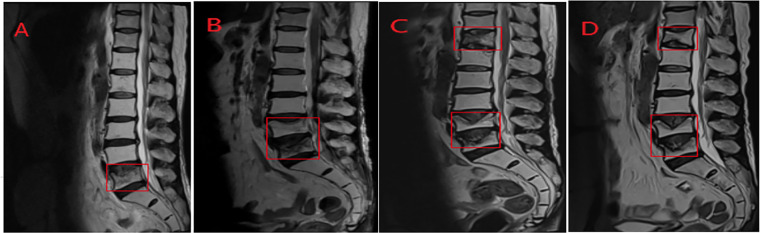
Lumbar Spine MRI scanning of the patient. (A) Compression Fracture of the L5 Vertebral Body. (B) Compression Fracture of the L4 and L5 Vertebral Bodies. (C) Compression Fracture of the T12, L4, and L5 Vertebral Bodies. (D) Bone destruction in the sacrum and the posterior part of bilateral iliac bones, and Compression Fracture of the T12, L4, and L5 Vertebral Bodies. Red box: Area of bone destruction.
